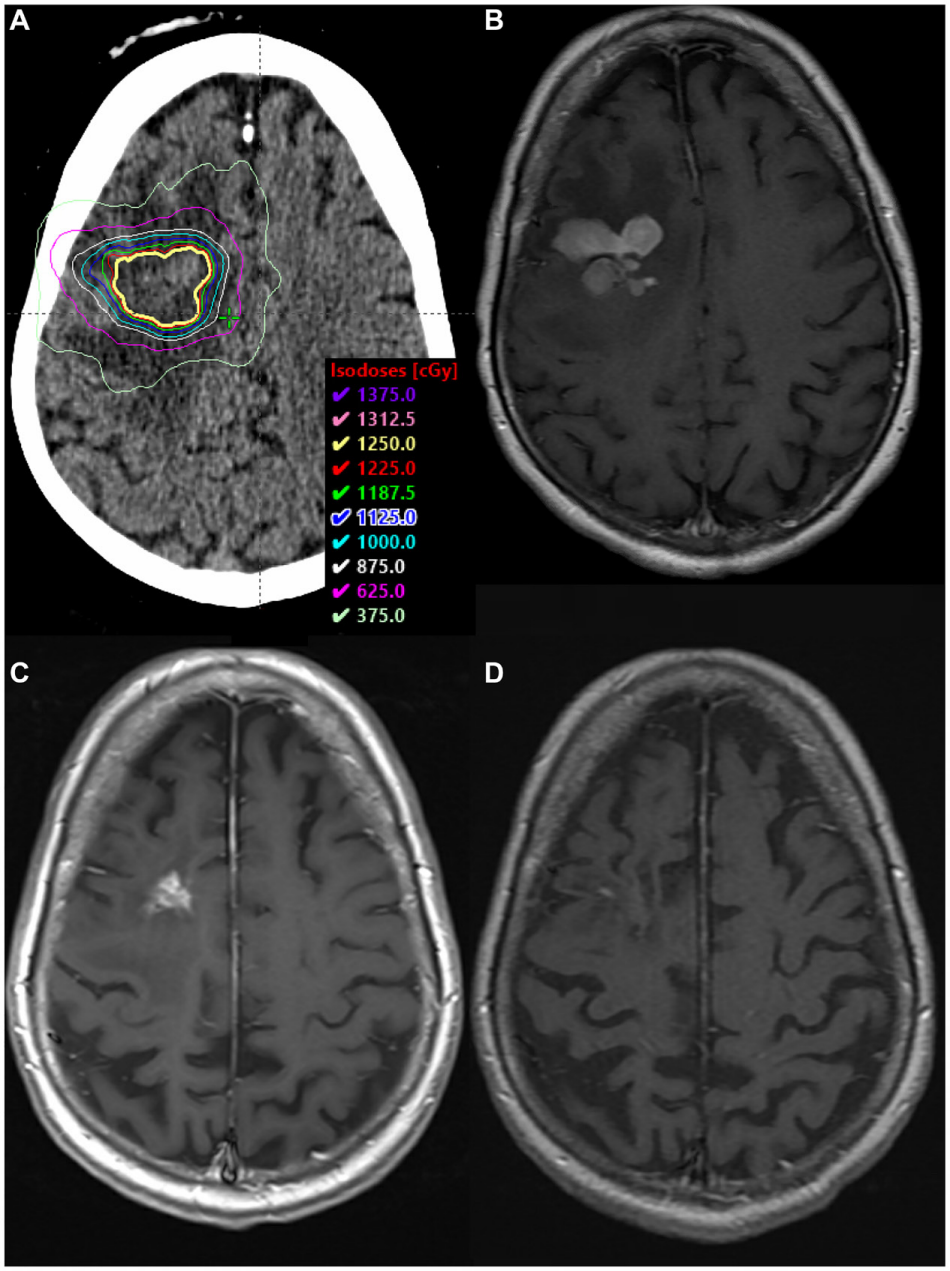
SRS boost treatment plan and MRI scans. (A) SRS boost treatment plan. (B) Enhancing residual disease prior to SRS boost on T1 sequence with contrast. (C) Biopsy-proven RN along the margin of the anterior body of the right lateral ventricle, within the SRS boost volume, on T1 sequence with contrast. (D) MRI >4 years after BV, demonstrating complete radiographic response on T1 sequence with contrast.
